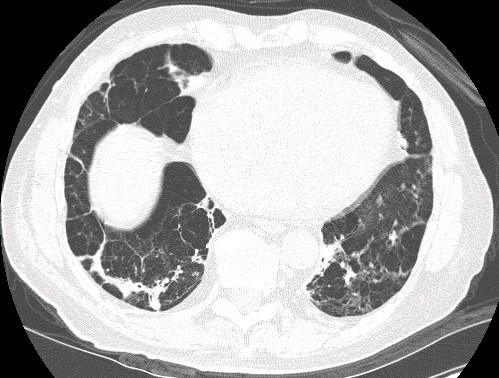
Single computed tomography image of the patient's chest. Bilateral lower-lobe emphysema with bullae is present, consistent with panlobular emphysema.
